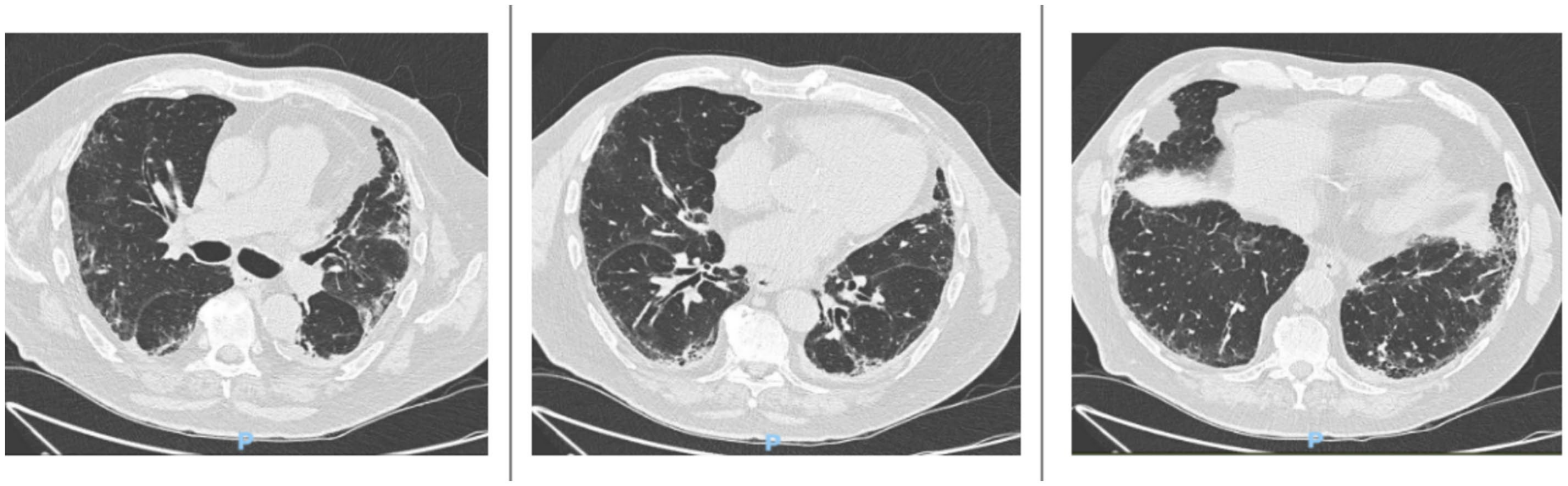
HRCT scans taken in November 2024 reveal a mild progression of interstitial lung disease (ILD). There is a slight increase in traction bronchiolectasis, which is more pronounced in the anterobasal and laterobasal segments of the left lower lobe, the anterior segment of the upper lobe, and the apical segment of the right lower lobe.
